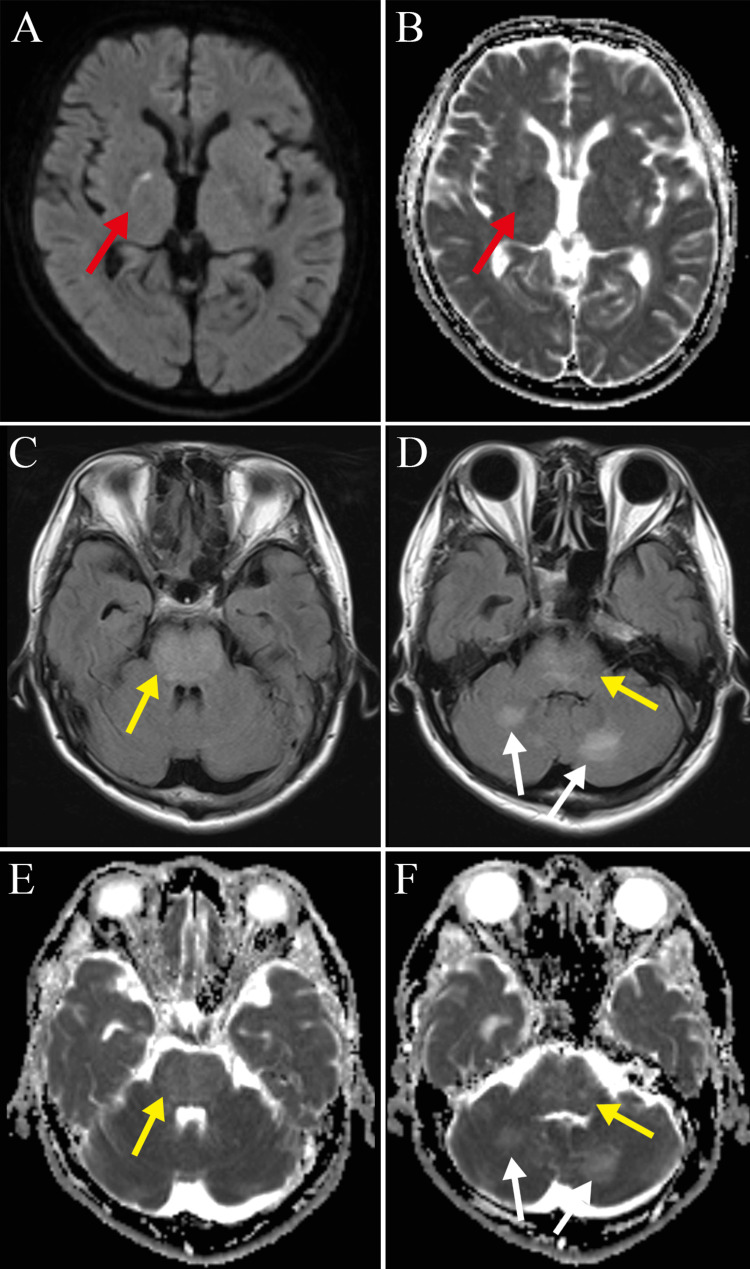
Cranial magnetic resonance imaging at admission (A,B) Diffusion-weighted imaging (DWI) shows a high signal, and the apparent diffusion coefficient (ADC) map shows a low signal extending from the right centrum semiovale to the posterior limb of the internal capsule (red arrows). (C,D) Fluid-attenuated inversion recovery (FLAIR) images and (E,F) the ADC map both show a high signal in the pons (yellow arrows) and bilateral cerebellar hemispheres (white arrows).
